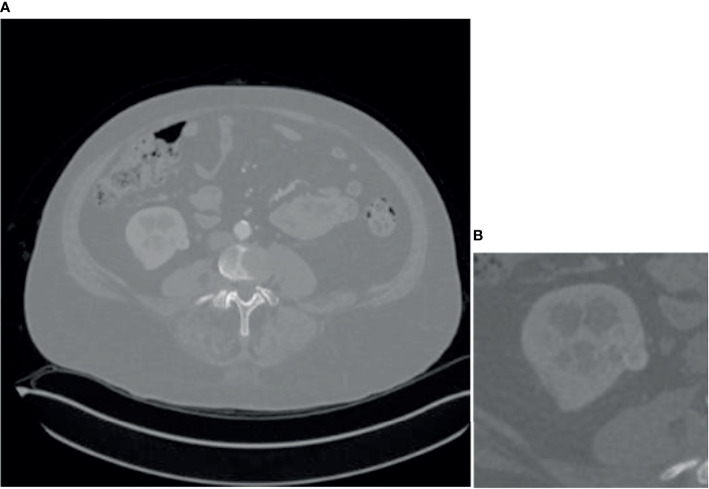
Result of coarse segmentation on CT image of 512 × 512 pixels to 128 × 128 pixels, (A) is CT image of 512 × 512 pixels, (B) is CT image of 128 × 128 pixels.
